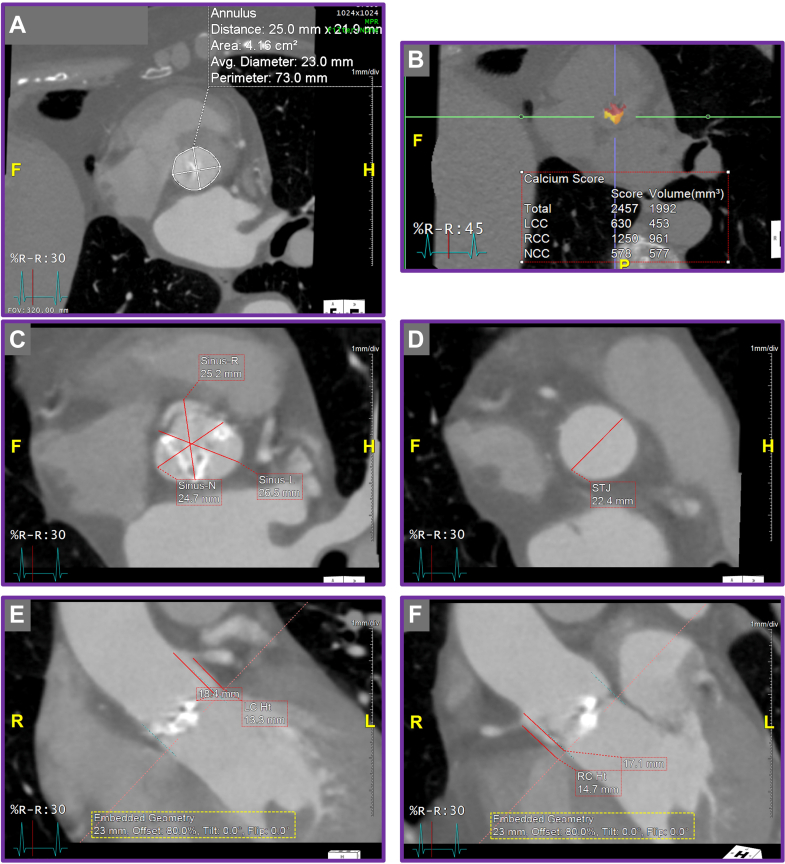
Initial Transcatheter Aortic Valve Replacement Protocol Computed Tomography (A) Annular measurements. (B) Predominant calcification on aortic valve leaflets. (C to F) Stovepipe annular configuration.
